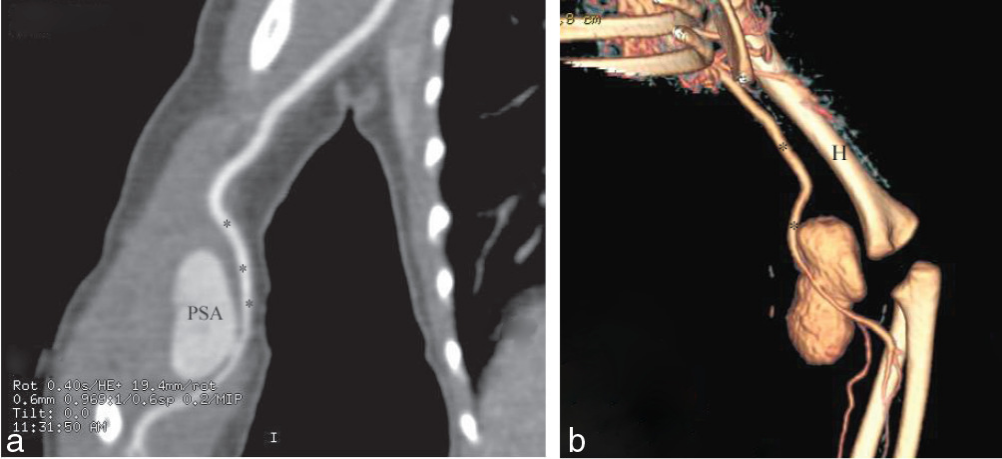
CT angiogram confirmed the sonographic findings. (a) On this longitudinal MIP reformat the PSA is seen displacing the brachial artery (*). (b) Three-dimensional reformat of the lesion performed for surgical planning showing its relationship with the brachial artery () and the humerus (H). MIP, maximum intensity projection; PSA, pseudoaneurysm.
